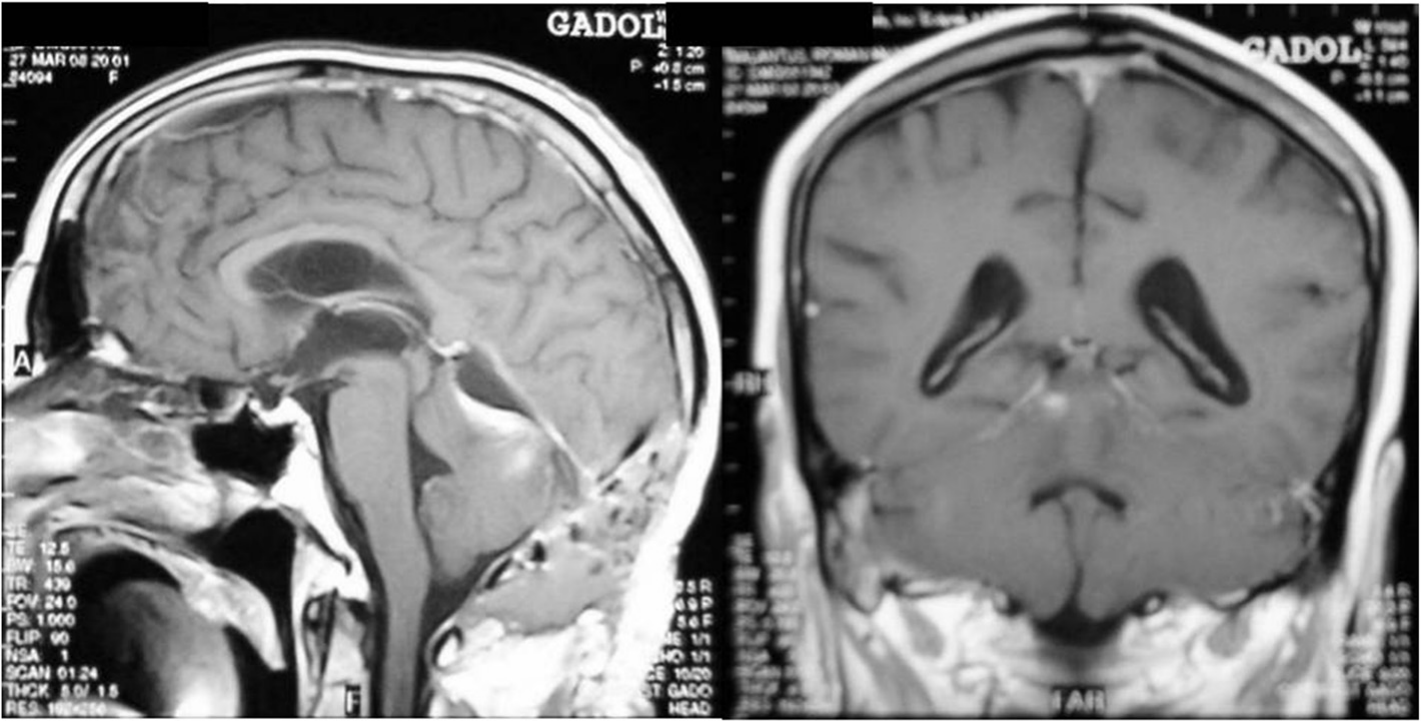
Postoperative gadolinium-enhanced T1-weighted sagittal and coronal magnetic resonance images showing complete mass resection
